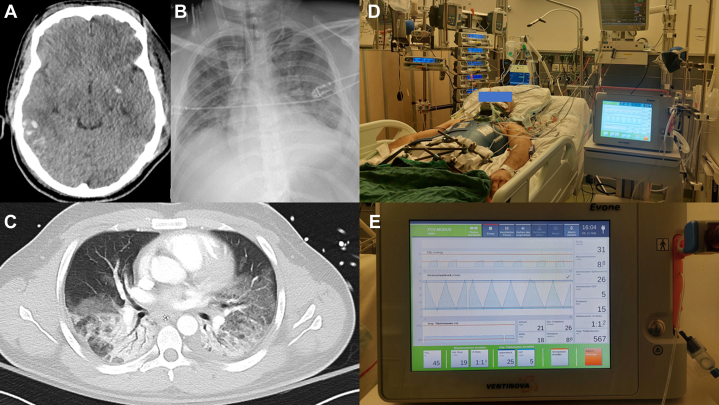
A-E, Imaging from the 30-year-old White male patient. A, Cerebral CT scan exhibiting brain edema, subarachnoid, and intracerebral hemorrhages. B, C, Chest radiograph (B) and CT scan (C) with features of ARDS. D, Photograph showing ICU setup. E, Photograph showing and flow-controlled ventilator (Evone; Ventinova Medical).
